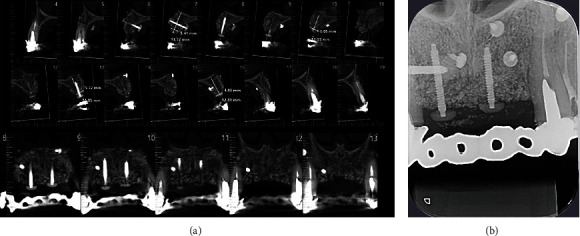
Tomographic (a) and periapical radiographic (b) images, showing improvement of bone contour and volume.
